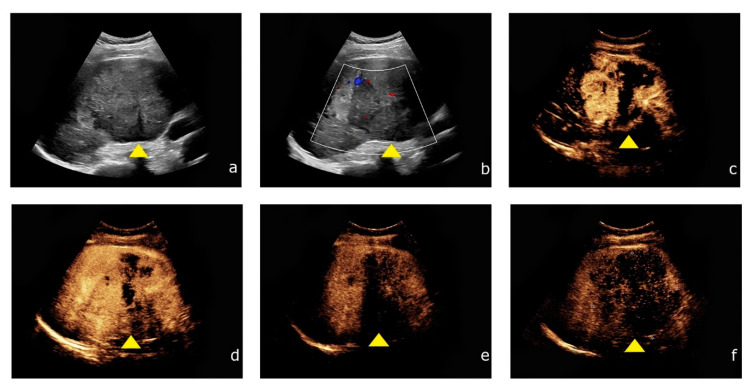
Contrast-enhanced ultrasound of HCC showing the typical contrast enhancement pattern. (a) Inhomogeneous liver lesion (yellow arrowhead) in the right liver lobe (8.7 cm) in native B-mode. (b) Intralesional hypervascularization is detected by Color Doppler. (c,d) After intravenous application of SonoVue^®^ (Bracco, Milan, Italy) the lesion shows rapid contrast enhancement during the arterial phase. (e,f) During the venous phase, the lesion shows typical wash-out during the venous phase.
